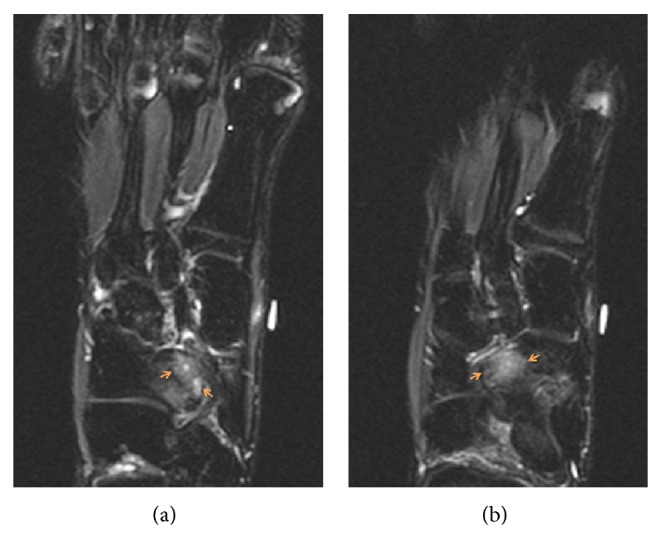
(a)-(b) Coronal short tau inversion recovery (STIR) unenhanced MR images of the right foot in a 17-year-old male with cuboid-navicular coalition demonstrate marrow edema and cystic change in the cuboid (arrows, (a)) as well as bone marrow edema in the navicular (arrows, (b)).
